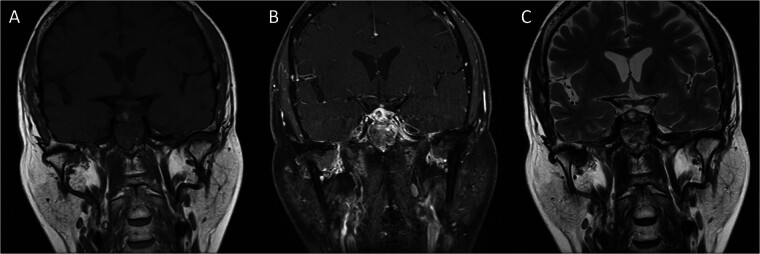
Postoperative brain MRI. Coronal T1WI (
